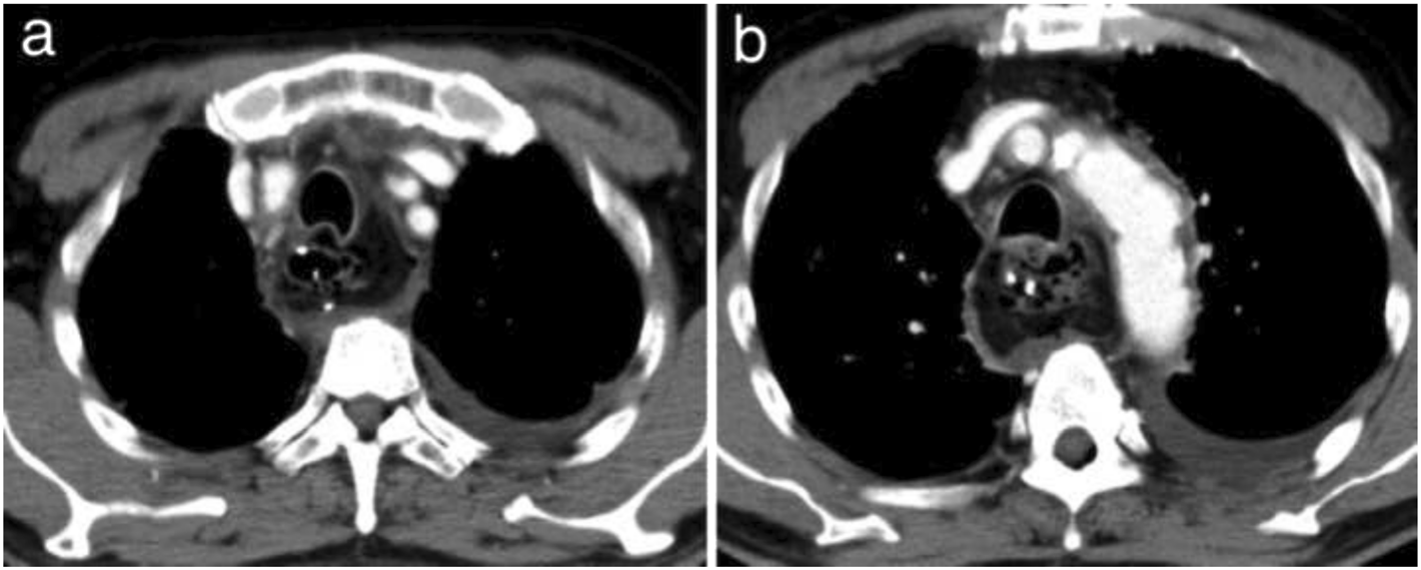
Chest contrast computed tomography findings. Contrast enhancement was significantly less in the wall at the fundus of the stomach and the vessels in the greater omentum (a). Pneumatosis intestinalis was observed in the gastric wall. According to the findings, this was diagnosed as gastric tube necrosis (b).
